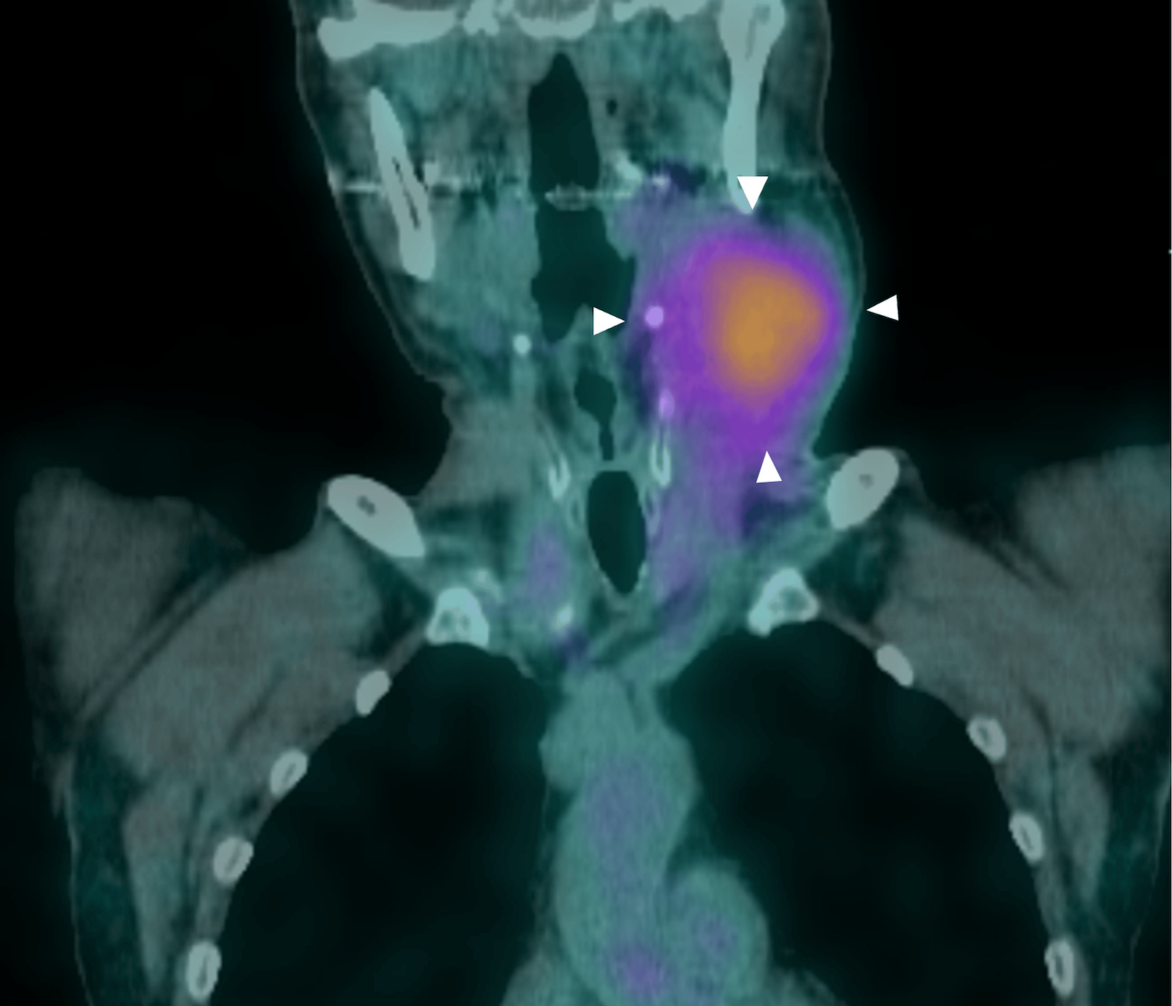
Somatostatin receptor scintigraphy Somatostatin receptor scintigraphy showing uptake in cervical lymph node metastasis (white triangles).
